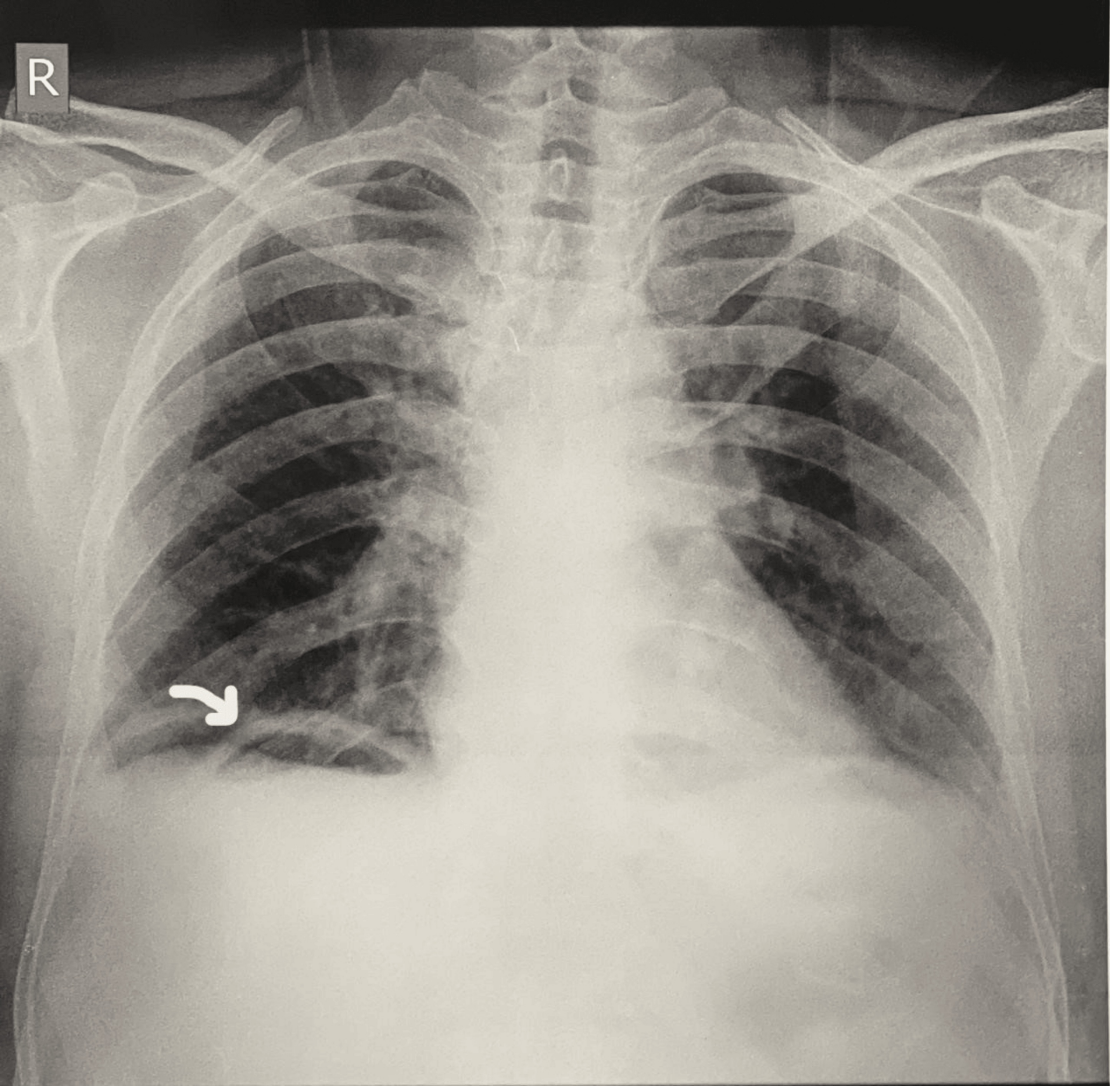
Chest X-ray (preoperative) Chest X-ray showing free air under the right dome of the diaphragm (white arrow)
